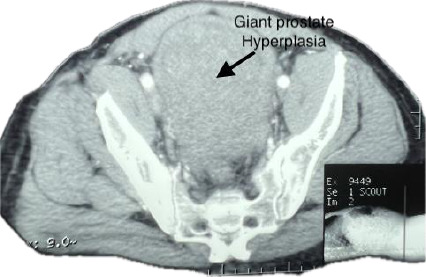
Pelvic CT scan showing giant hyperplasia of the prostate, estimated weight 541g (10.2 × 8.3 × 12.3 cm)
